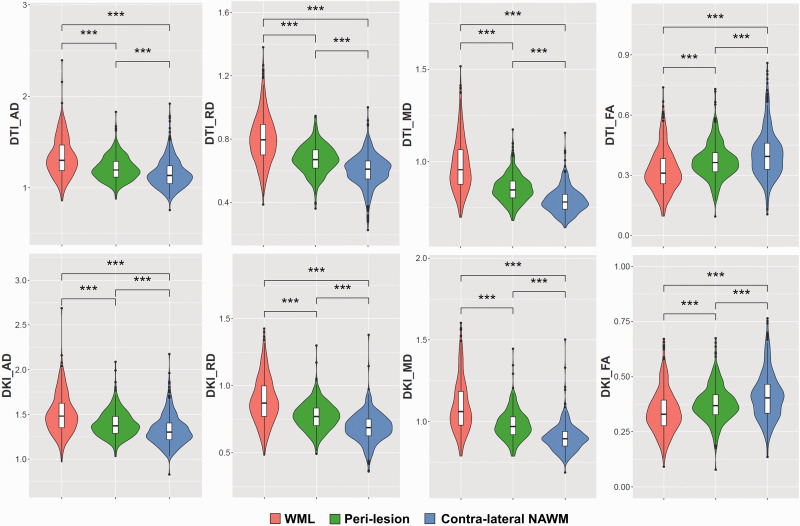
Heuristic diffusion MRI parameters.
